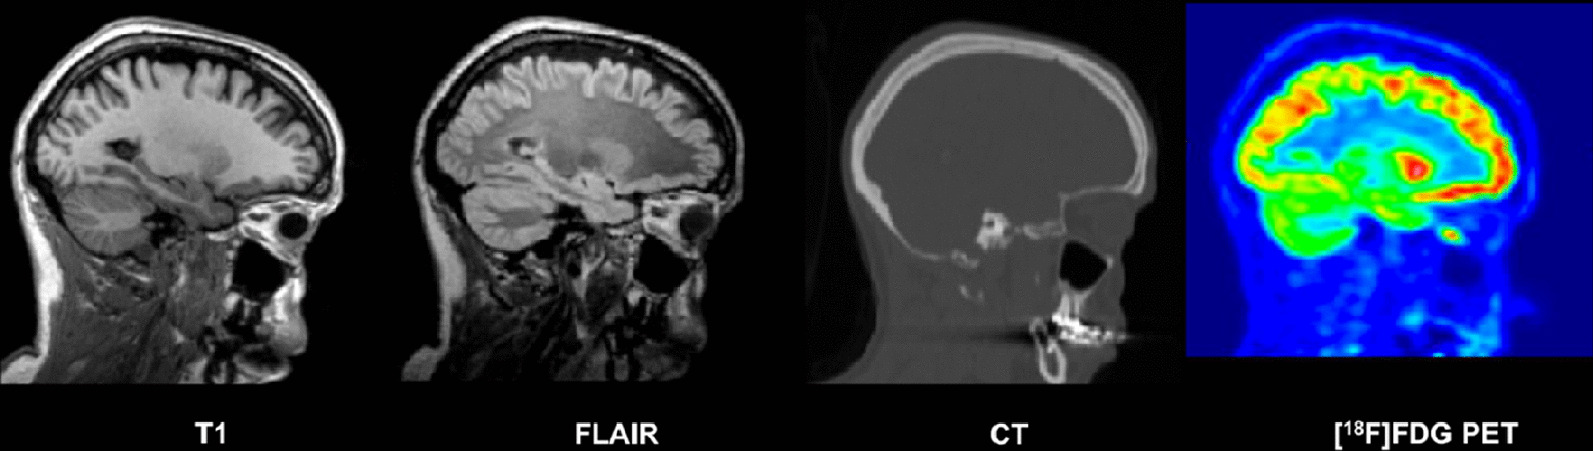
Example of coregistered T1 MRI, FLAIR MRI, CT and [^18^F]FDG PET images (sagittal plane) for one subject of the database
